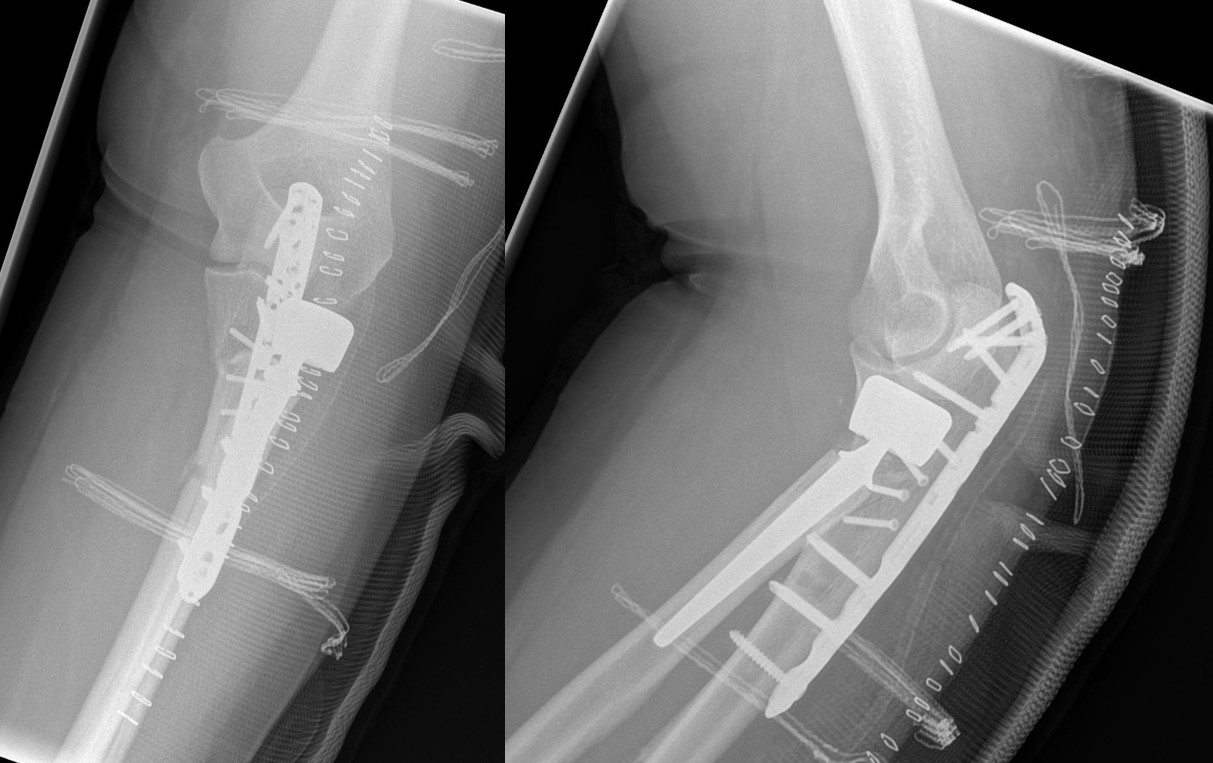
Postoperative radiographs. Osteosynthesis of the ulna was performed using a proximally precontoured olecranon plate applied to the posterior surface of the ulna. Implantation of a cemented bipolar radial head prosthesis.
